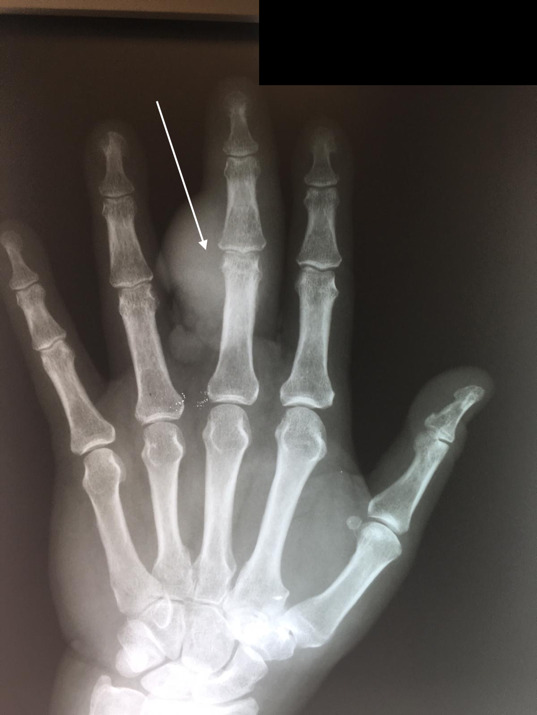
PA radiograph of left hand revealing the radiopaque lesion on the ulnar aspect of the third digit, centered at the PIP joint.
